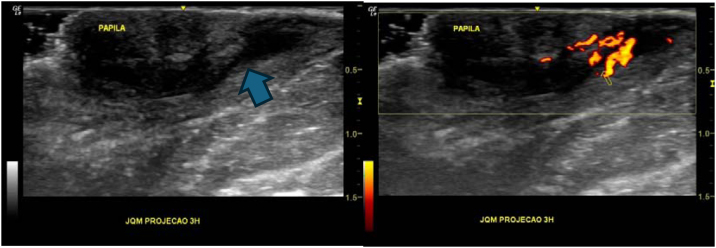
Ultrasound findings: Areolar HS – Fistula in the dermis/hypodermis at the junction of the medial quadrants of the right breast, periareolar, in a 3H projection with communication with a duct in the retroareolar region (blue arrow). Increased locoregional vascularization is noted on Doppler (Power) study.
